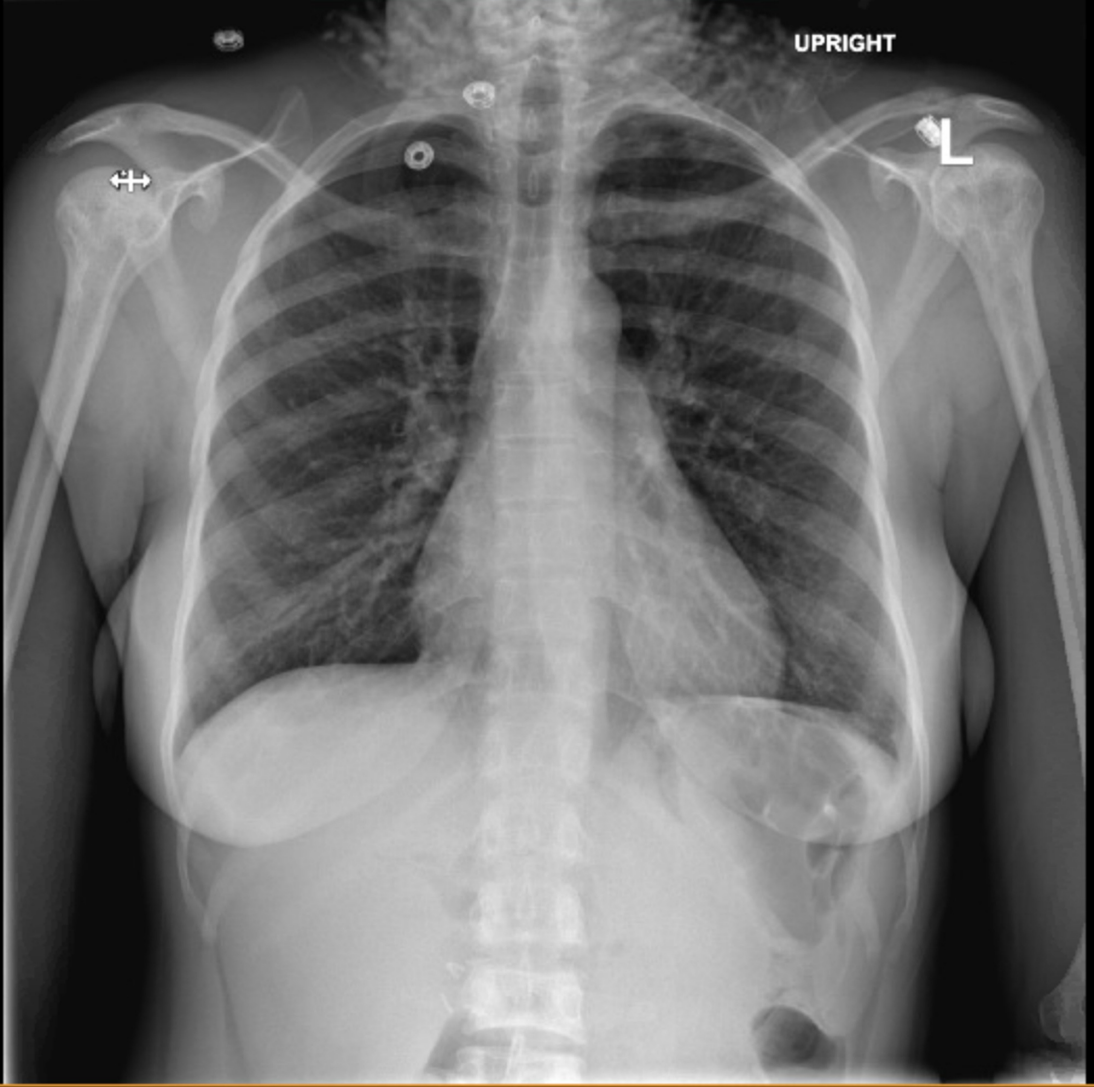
Patient’s chest X-ray on admission
